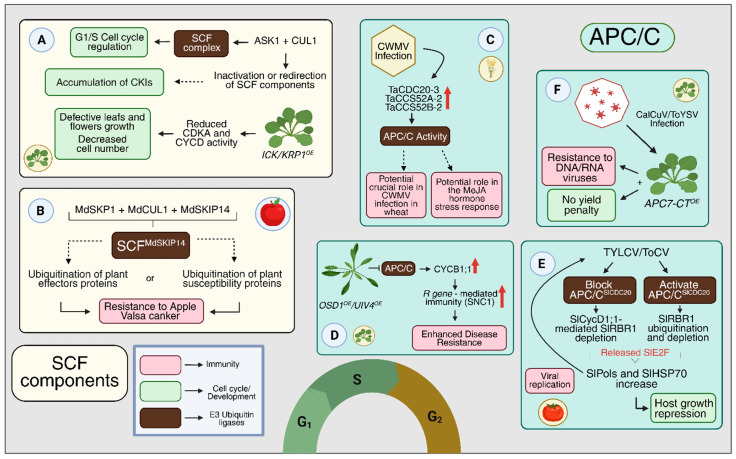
Integration of cell cycle regulation and immunity by ubiquitin-mediated pathways in plants. (A) Immune activation generates competition for the components (ASK1 and CUL1) leading to inactivation of the SCF^SKP2A/B^ complex and accumulation of ICK/KRP1, which reduces CDKA/CYCD activity. (B) The SCF^SKIP14^ complex (Md*SKP1*, Md*CUL1*, and Md*SKIP14*), promoting immune responses and resistance to Infection by *Valsa mali*. (C) CWMV infection in wheat increases the expression of APC/C regulators (Ta*CDC20-3* and Ta*CCS52*). (D) Inhibition of APC/C by OSD1 or UVI4 leads to CYCB1;1 accumulation, activating *R* gene-mediated immunity, such as *SNC1* and increasing resistance to *Pseudomonas syringae*. (E) TYLCV and ToCV viruses sequester APC/C in tomato plants, resulting in reduced growth. (F) Overexpression of *APC7-CT* confers resistance to DNA and RNA viruses without reducing plant productivity. The functional roles of each component are described in detail in the text and Table 2. The black arrows indicate regulatory outcomes or downstream consequences of the indicated molecular events. Created in BioRender. Köhn carneiro, A. (2026) https://BioRender.com/yqsb4x1 (accessed on 1 February 2026).
